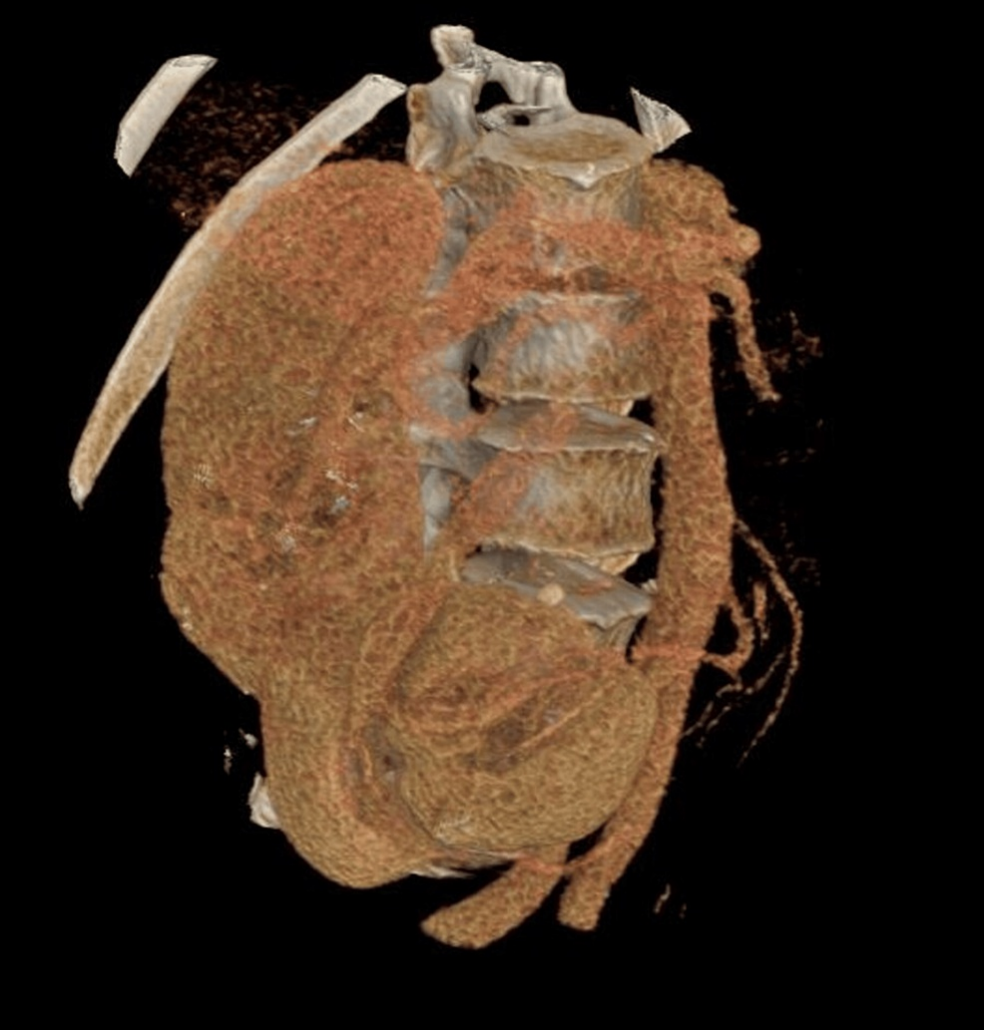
Volume rendering reformatted image of arterial phase computed tomography (CT) showing the S-shaped kidney. The kidney, its vasculature, and the lumbar spine were segmented based on density. The increased cranio-caudal length and the rotation of the kidney can be appreciated.
